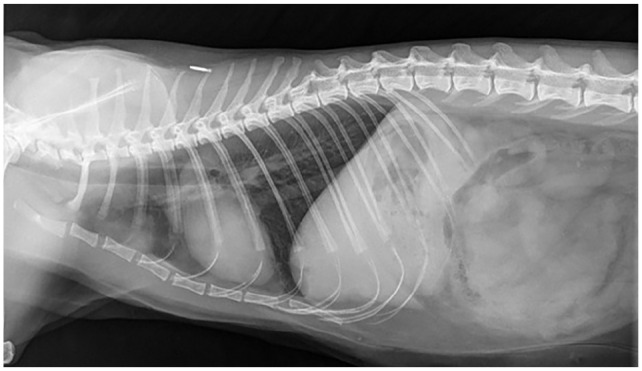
Left lateral thoracic radiograph showing sternal and tracheobronchial
